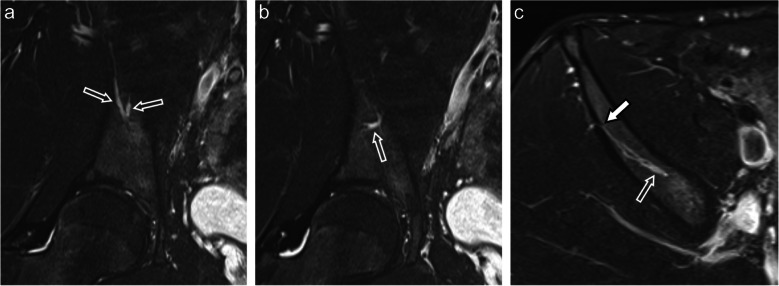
MRI of the right ilium bone in a 25-year-old male showing the peripheral branches of a CVC. a, b Several prominent branches (outline arrows) can be seen in two adjacent coronal STIR images. c On the axial STIR image two branches of the CVC show an almost parallel course (outline arrow), and the exit point of anterior branch (white arrow) can be seen on the lateral surface of the ilium bone, forming an anastomosis with the superior gluteal artery. STIR: short tau inversion recovery
